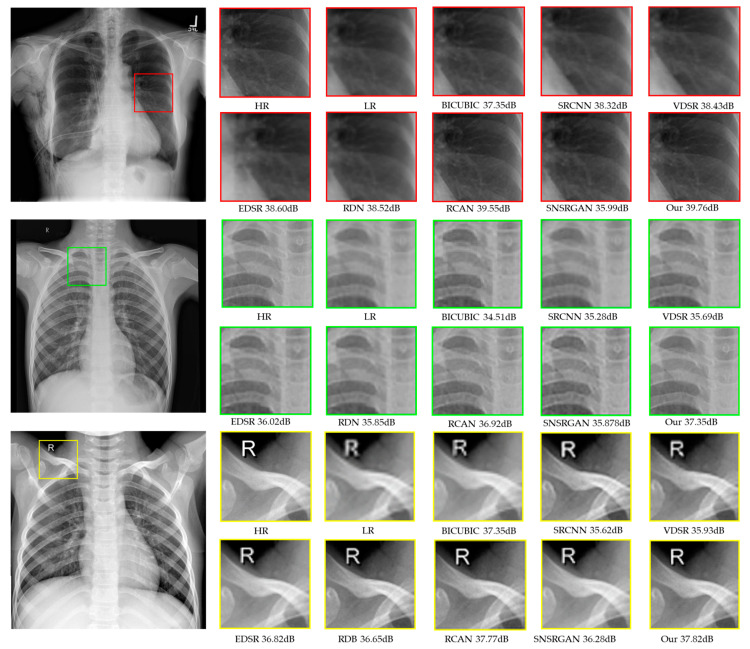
Visual comparison of super-resolved (up-scaled by a factor of 4×) chest X-ray images from the CXR1 (red squares), CXR2 (green squares), and CXR3 (yellow squares) datasets. The HR images generated through traditional interpolation methods, other SOTA methods, and our proposed model are presented.
